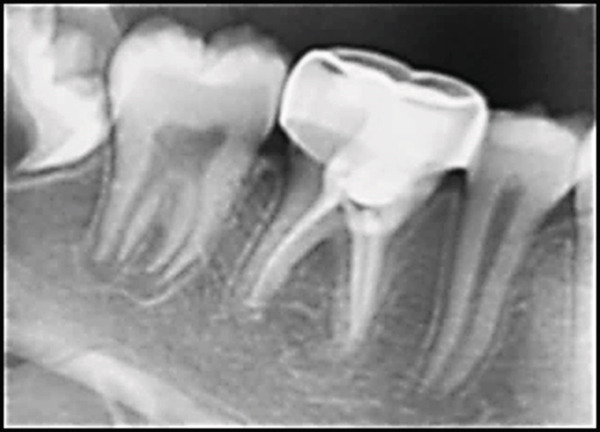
Postoperative radiograph of 46.
